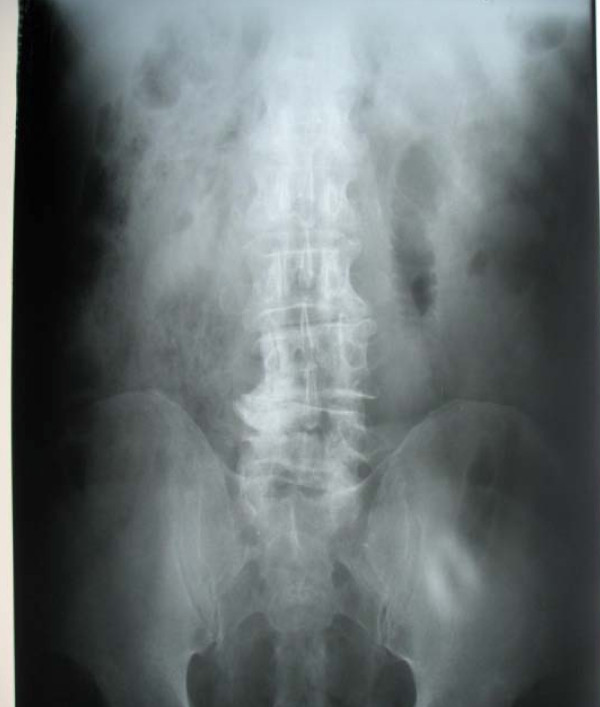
Supine abdominal X-ray showing dilated jejunal loop on the left side.
